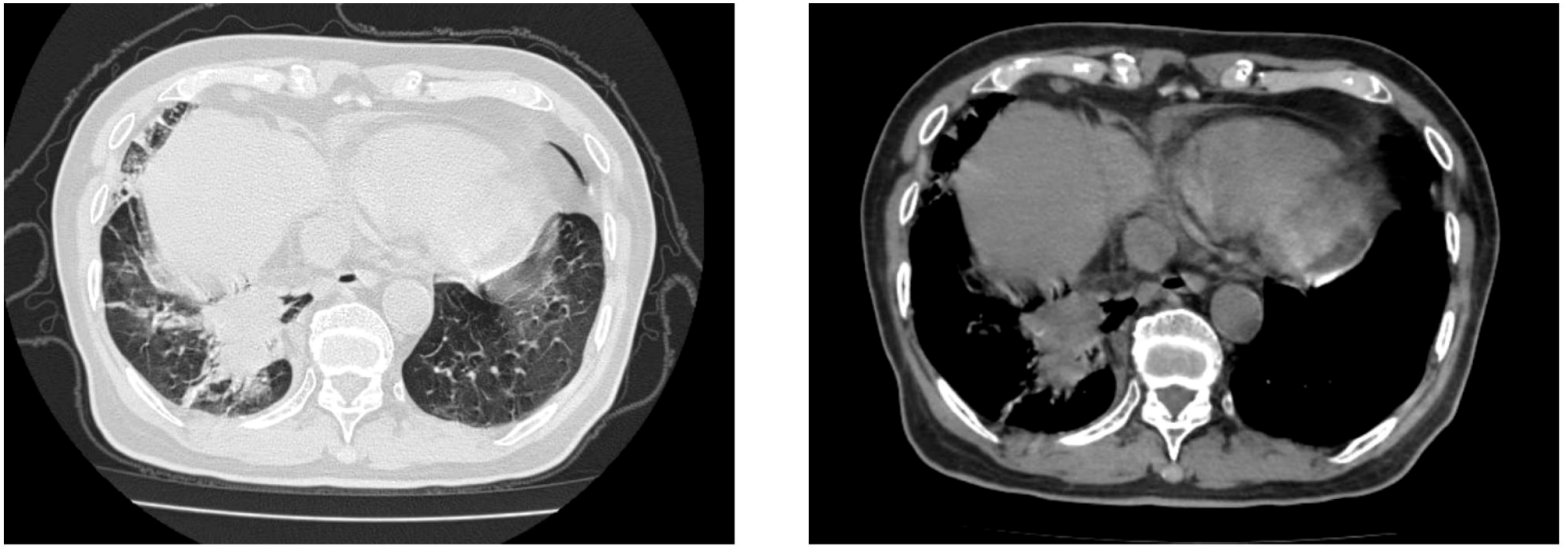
Chest computed tomography (CT) scan revealed a lobulated mass in the posterior basal segment of the right lower lobe, accompanied by partial subsegmental bronchial obstruction and distal inflammatory changes. Additionally, an increased soft tissue density was observed in the right lower hilar region.
